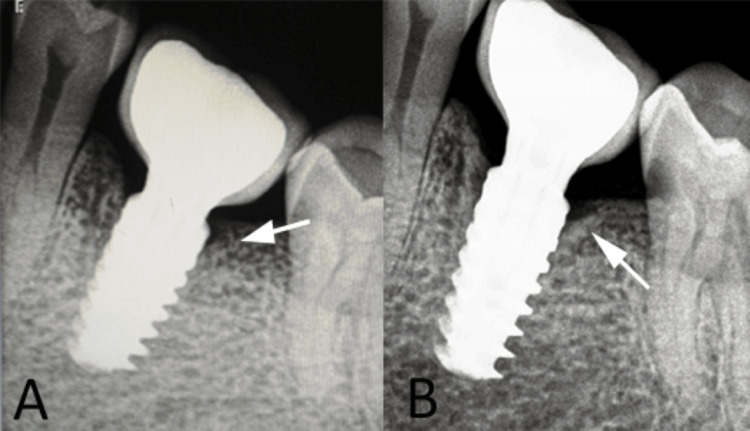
Follow-up evaluation findings A: post-loading radiograph showing crestal bone levels around the immediate implant (white arrow); B: three-year follow-up radiograph demonstrating minimal to mild marginal bone loss (white arrow). Original patient images, used with the patient's permission.
